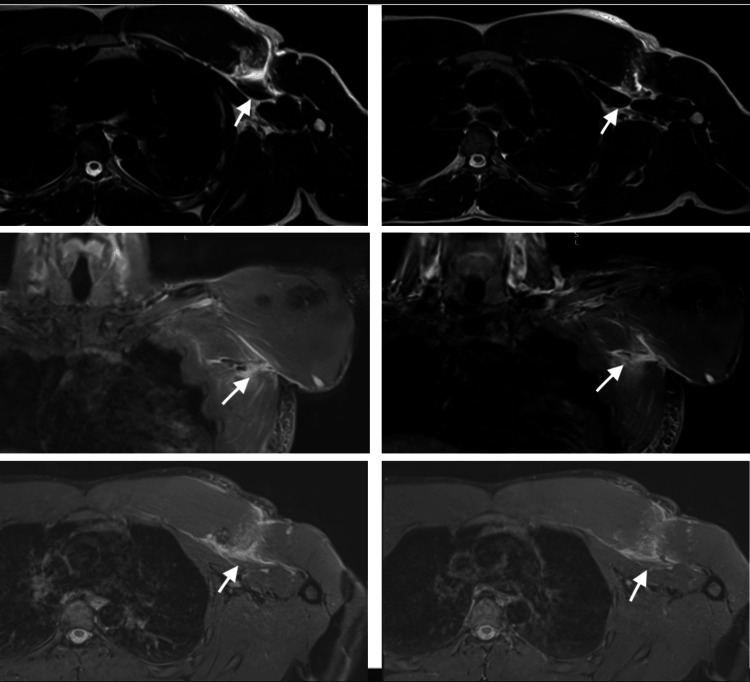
MRI scan of the left chest showing a rupture at the myotendinous junction of the sternal head of pectoralis major with retraction and associated diffuse edema in the involved muscle and large hematoma in the gap between the humerus and the retracted muscle segments. The transverse measurement of the tear gap was 4.5 cm with an intact tendinous bony insertion measured length of 2.5 cm.
